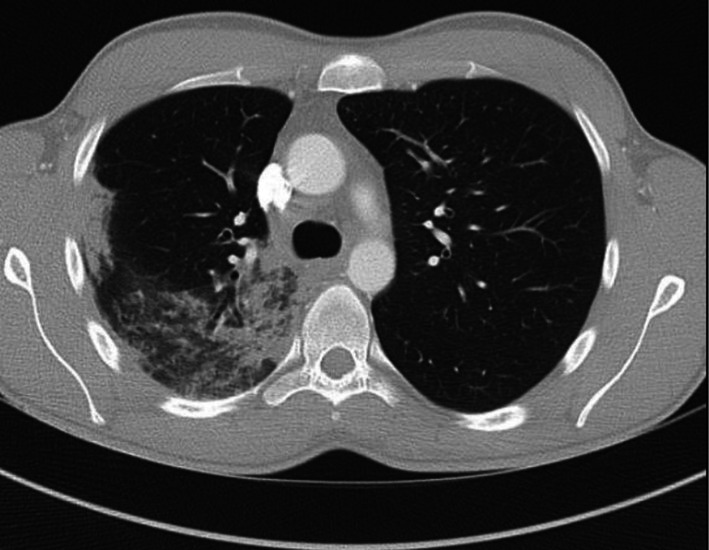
CT pulmonary angiogram demonstrates bilateral subpleural ground‐glass opacities with patchy consolidation. The peripheral distribution is characteristic of eosinophilic pneumonitis and hypersensitivity‐type lung injury rather than infection.
